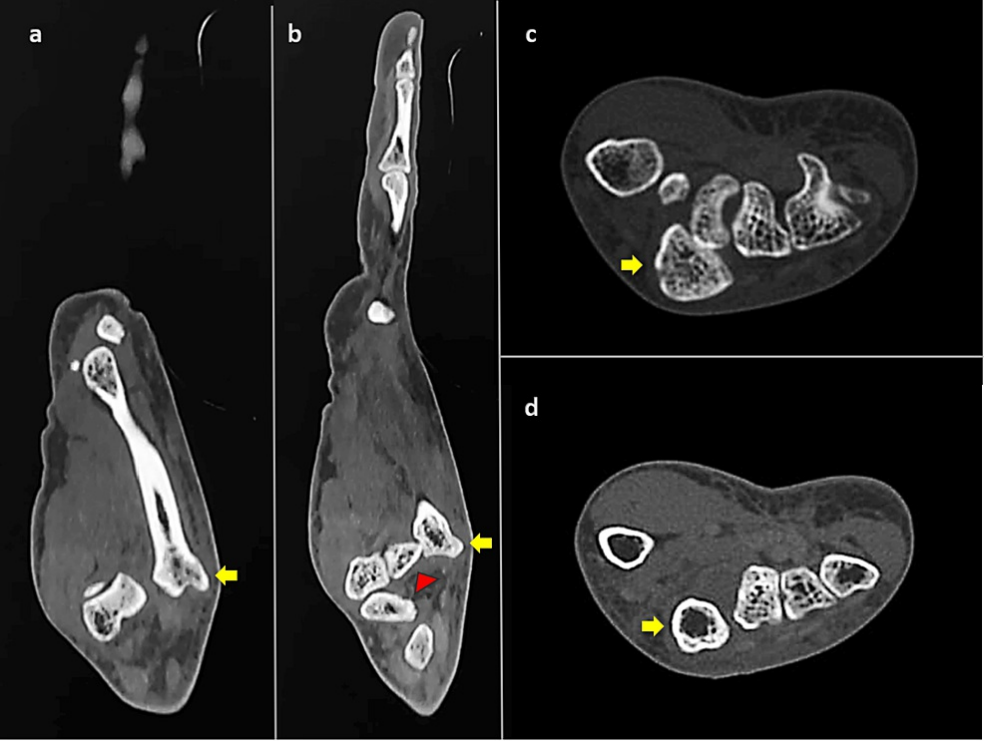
Computed tomography of the hand (a) and (b) sagittal computed tomography sections demonstrating dorsal dislocation of the second metacarpal. Yellow arrow indicates dorsal dislocation of the base of the second metacarpal, while the red arrowhead indicates the trapezoid bone. (c) and (d) axial computed tomography sections showing disruption of the transverse metacarpal arch. Yellow arrow indicates the second metacarpal, which is no longer within the arch.
